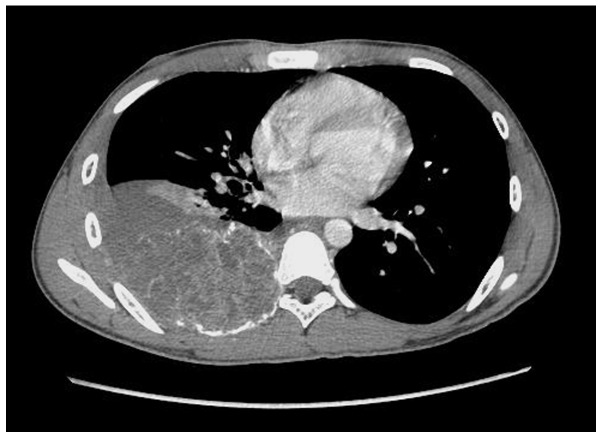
Computed tomography scan showing an irregularly shaped 12×10×10-cm expansion mass beside the 7th thoracic vertebra. Disappearance of the cortex and lytic changes in the 7th ribs are demonstrated. Atelectasis of the right lower lobe is also evident.
